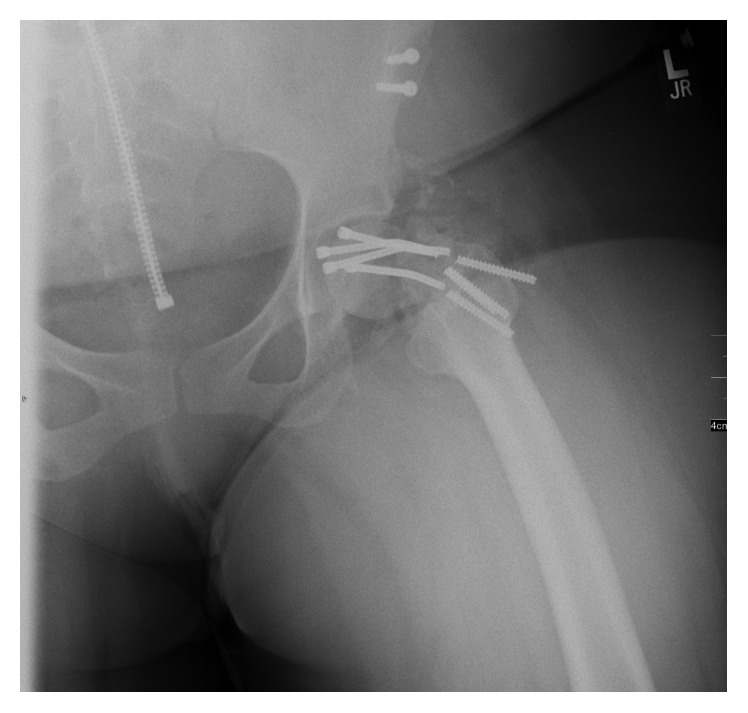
Anteroposterior radiograph demonstrating hardware fatigue and breaking of screws.
